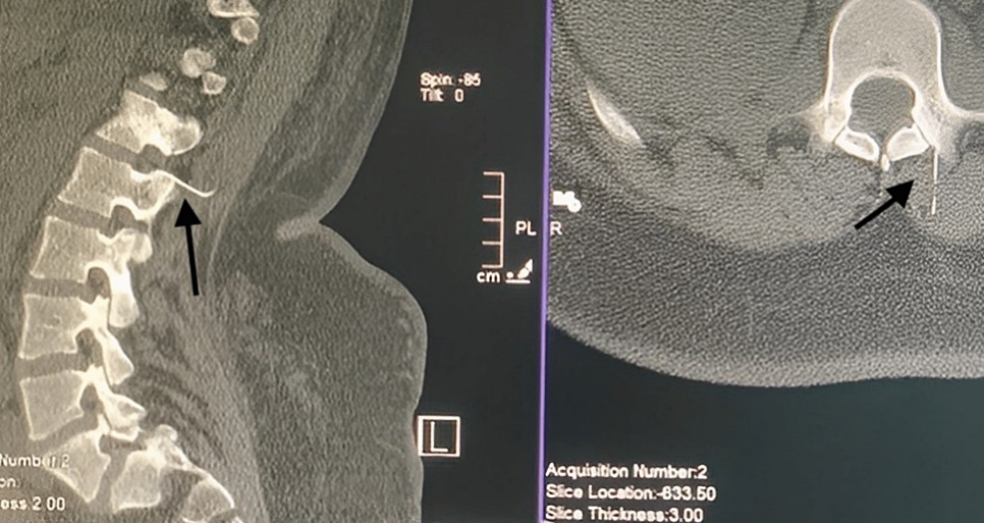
CT scan thoraco-lumbar spine axial and sagittal views show the broken needle tip at the left intervertebral foramen between T12-L1
